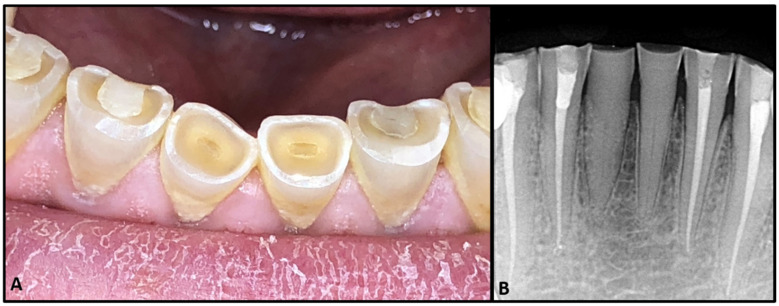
(A) Image showing severe tissue loss due to severe bruxism. (B) Initial pre-op X-Ray.
